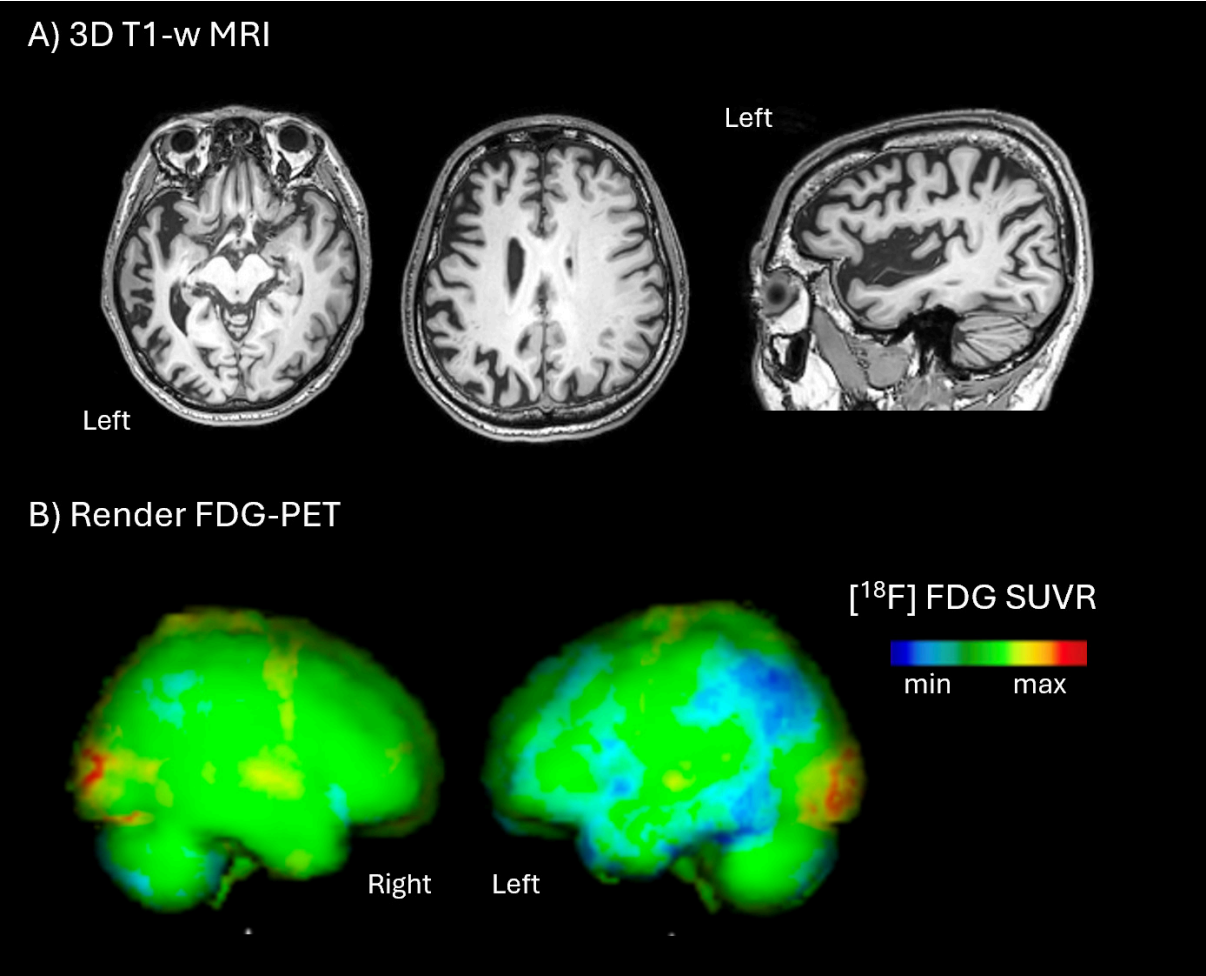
Imaging. (A) T1-weighted MRI demonstrates predominantly left-sided atrophy in frontal and temporo-parietal regions. (B) PET FDG shows hypometabolism (blue areas) in the left posterior temporo-parietal and inferior frontal cortices.
